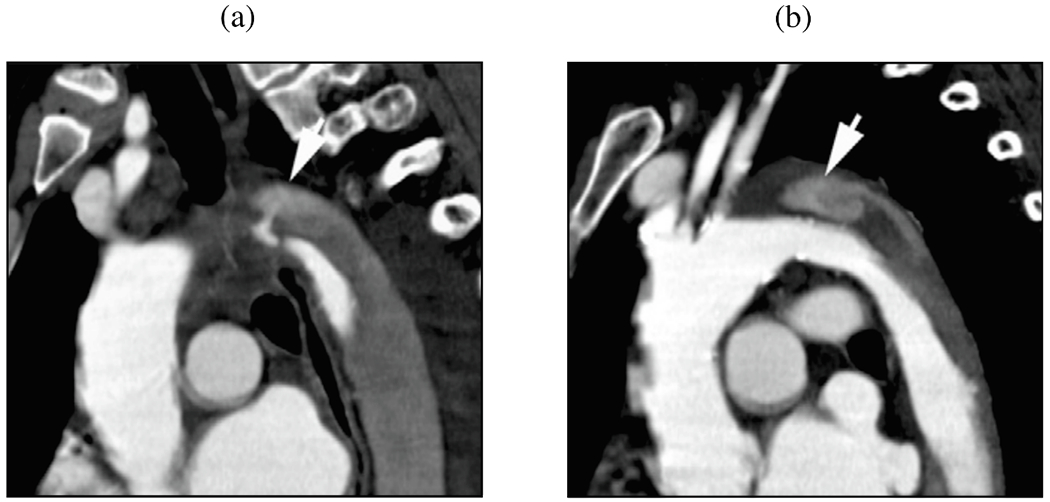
Two phases of imaging after contrast injection, (a) and (b), of enhanced CT images showing a sac-like formation of partially thrombosed false lumen, defined as a blind pouch with a persistent entry tear (arrows). Figure is reproduced from Sueyoshi et al. [327] with permission from Elsevier.
